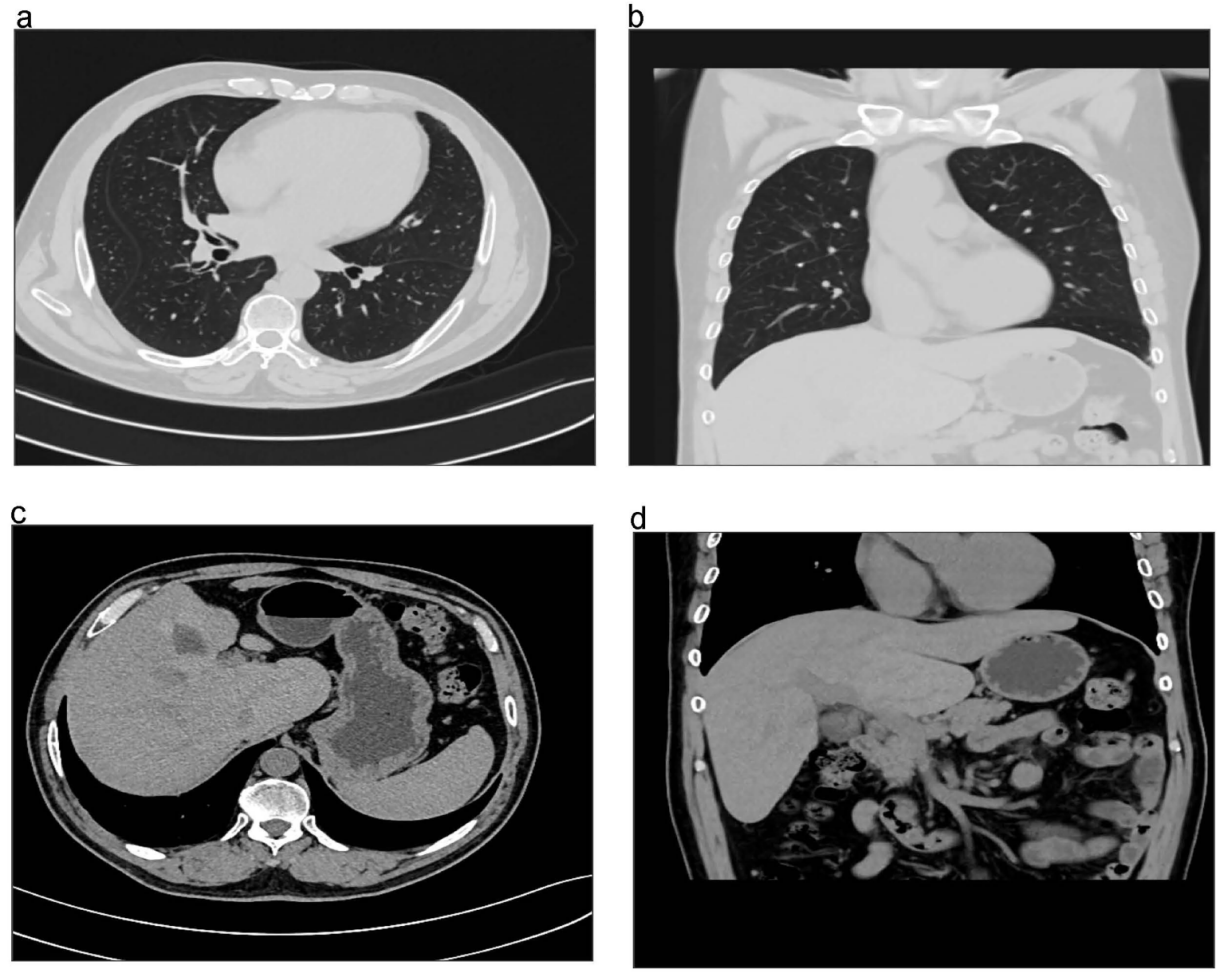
A repeat CT performed three months after discharge from the hospital showed resolution of lung and liver infections. (a-d) The CT images demonstrate the successful resolution of the infections in both the lung and liver, indicating a positive outcome of the treatment
